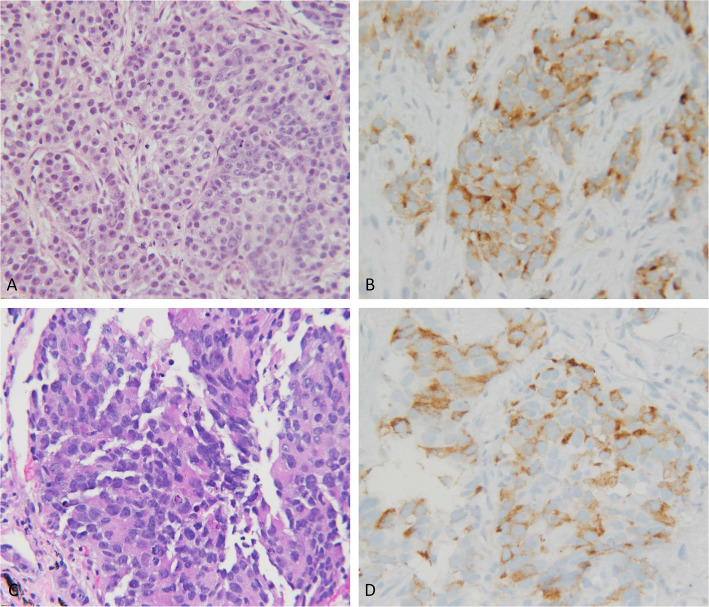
Prostate adenocarcinoma showed PSMA positivity in tumor cell pattern. a. Primary prostate adenocarcinoma (PSMA, 200x); b. Metastatic prostate adenocarcinoma (PSMA, 200x); c, d. Metastatic prostate adenocarcinoma (C. HE, 200x; D. PSMA, 200x)
